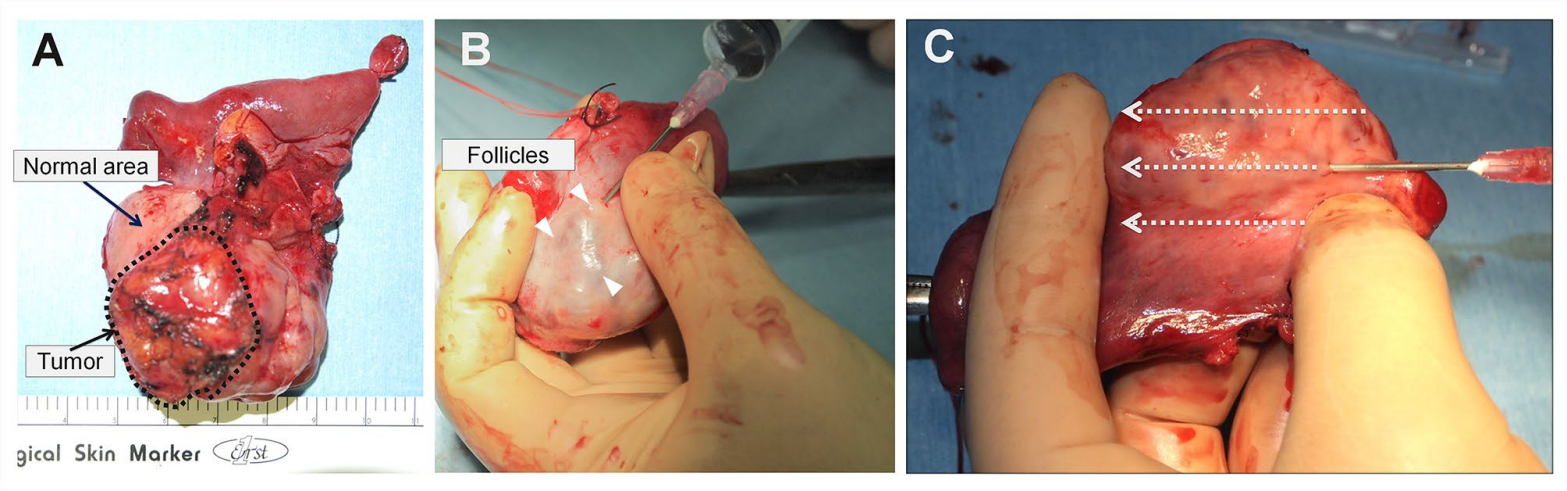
Oocytes retrieval from resected ovary. A: The tumor area was identifiable by palpation and ultrasound. B: Multiple follicles were observed on the opposite side of the tumor, and visible follicles were first punctured. C: The entire ovarian cortex was punctured, even in areas where follicles were not visible
